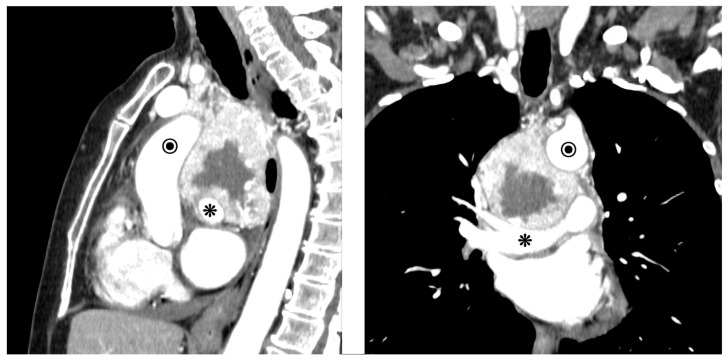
Coronal and sagittal computed tomography images showing a well-defined posterior mediastinal paraganglioma, surrounding pulmonary artery (asterisk) and ascending aorta (encircled) [Original images based on the authors’ personal surgical experience].
